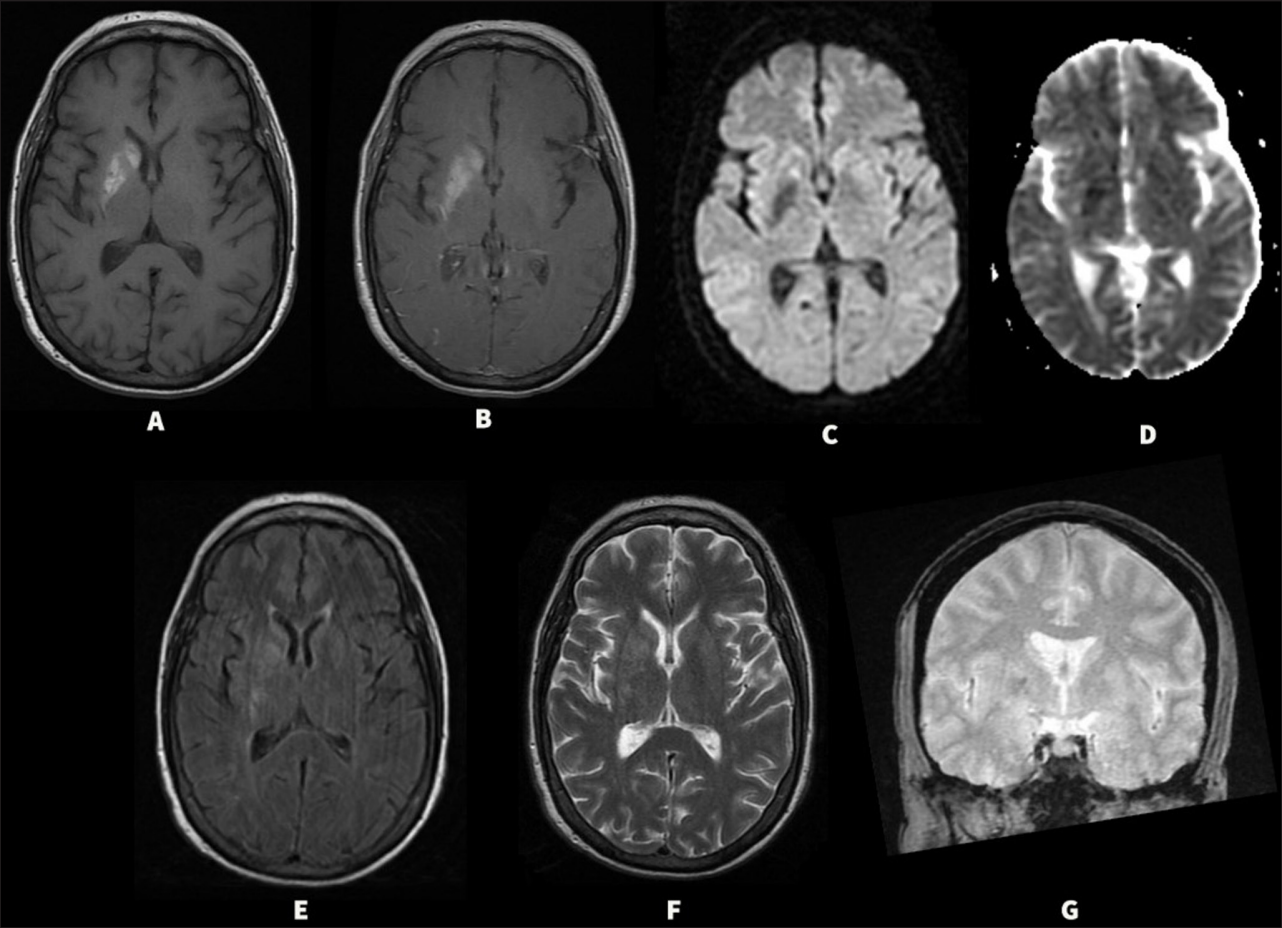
(Case 1) Initial MRI showing ill-defined T1 hyperintensities in the right basal ganglia (A) without changes in the internal capsule and minimal contrast enhancement with gadolinium without surrounding edema (B). Diffusion weighted sequences showing decreased signal in the right basal ganglia with no restriction (C & D). Fluid attenuated inversion recovery (FLAIR) images show isointense signal (E) with hypointenisty on T2 images (F) in the basal ganglia. There was no significant gradient echo (GRE) susceptibility to suggest hemorrhage (G).
